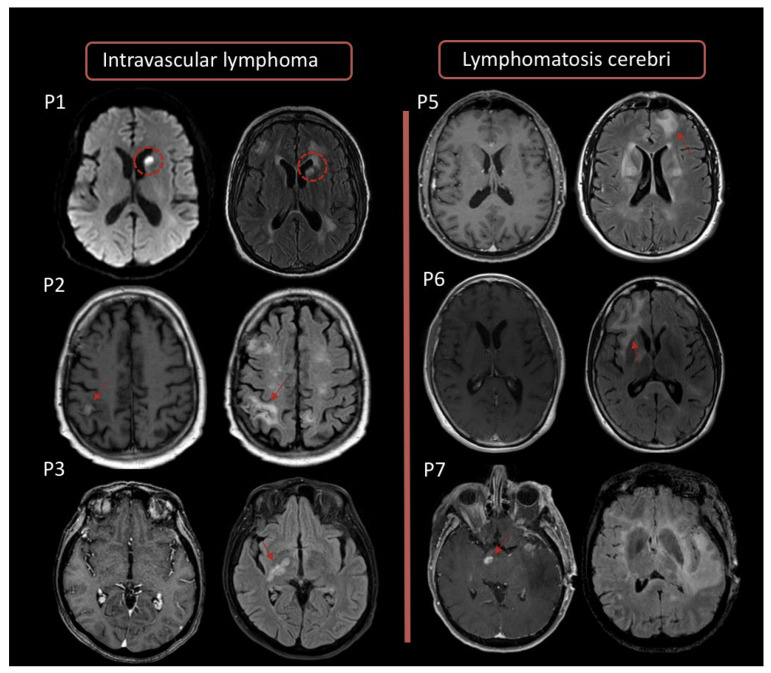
Case examples of MRI presentations of intravascular lymphoma (Patients 1, 2, and 3) and cerebral lymphomatosis (Patients 5, 6, and 7). Left: intravascular lymphoma presents as cortical, subcortical, or periventricular lesions with FLAIR hypersignal (2nd column) and little or no gadolinium-weighted T1+ enhancement. The diffusion-weighted image of the first patient (P1) shows a high hypersignal of the head of the caudate nucleus mimicking a stroke (red dotted circle). Right: lesions of cerebral lymphomatosis appear as infiltrating, confluent lesions with FLAIR hypersignal, in the deep and subcortical white matter (red arrows), without mass effect (4th column). T1+ gadolinium-weighted images (3rd column) show no enhancement, with the exception of the last patient (bottom right) who had both typical homogeneous enhanced lesions and an infiltrating FLAIR hypersignal.
